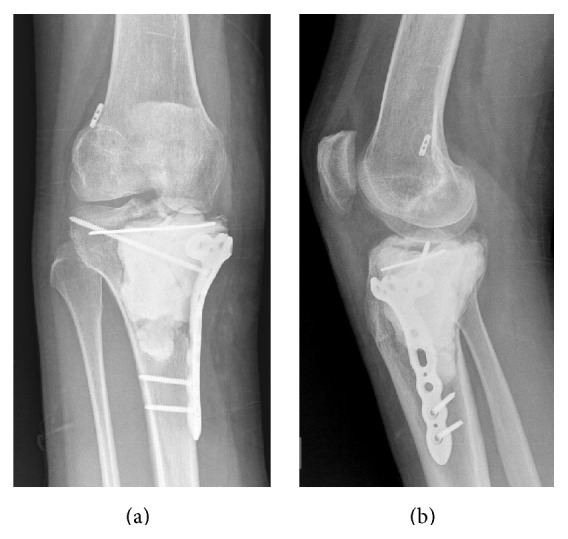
AP and lateral standing radiographs 4 months after giant cell tumor curettage and packing demonstrate stable postoperative change.
